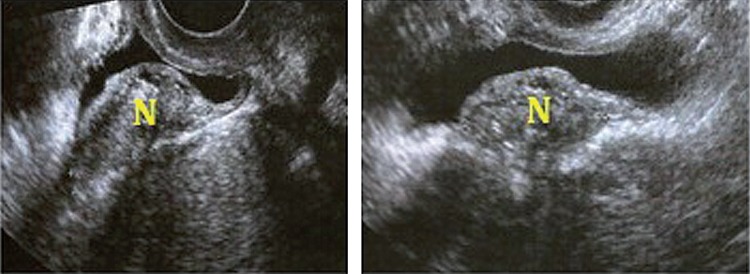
Transvaginal ultrasound with endometriotic endovesical lesion. (N)
